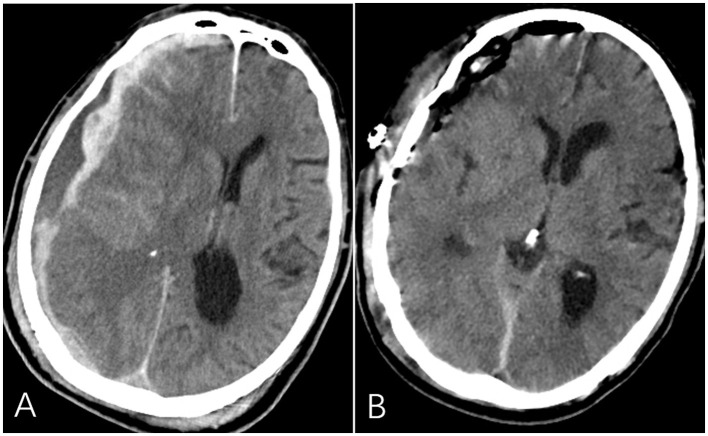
Pre- and post-operative findings on head computed tomography (CT) scans. (A) A massive traumatic subdural hematoma measuring 20 mm in thickness on the right frontotemporoparietal regions was shown on the preoperative head CT scan. (B) The subdural hematoma was completely removed through an emergency craniotomy.
